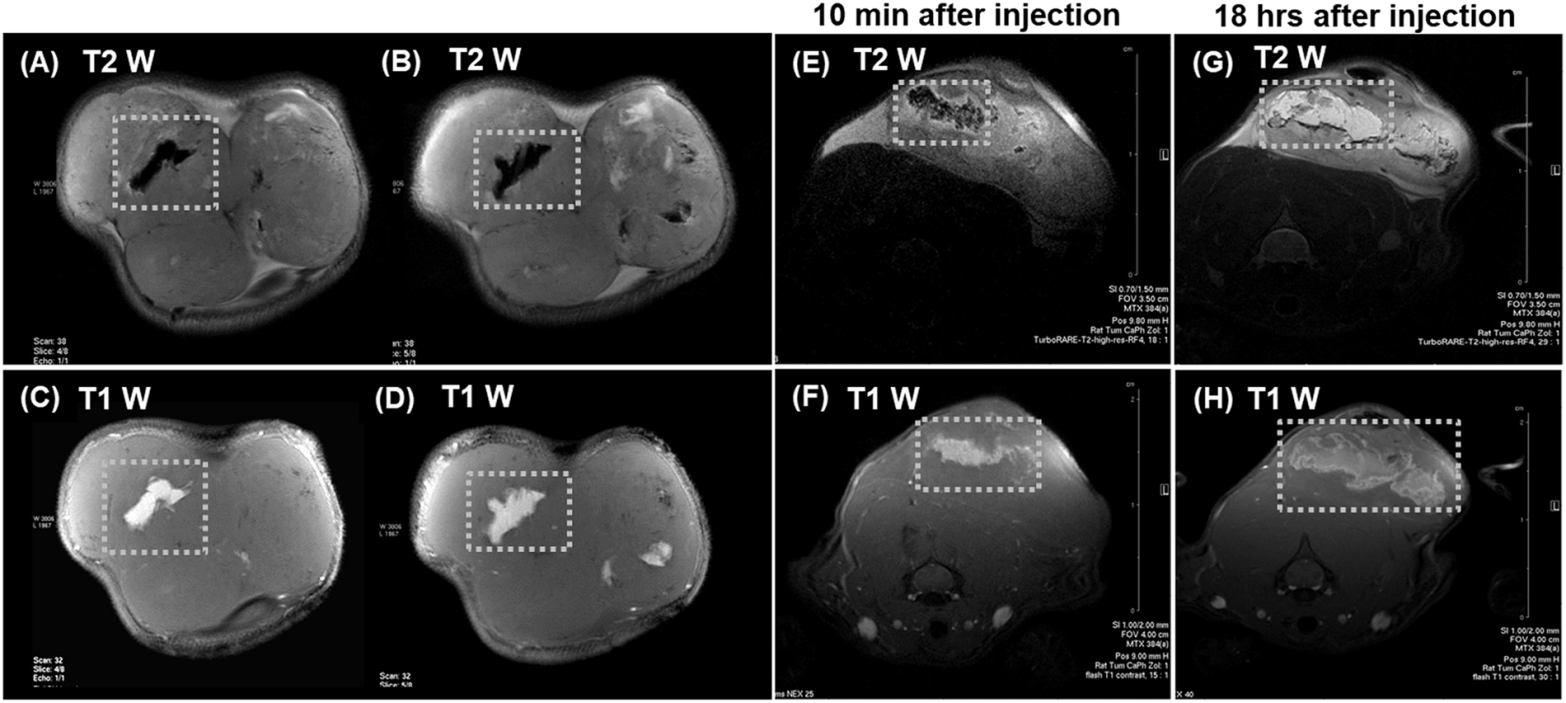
Dual T1-T2 contrast variation after intra-tumoral injection. (A,B) T2 weighted and (C,D) T1 weighted MR image (coronal section) of subcutaneous tumor after intratumoral nCP:Fe injection. (E) T2 weighted and (F) T1 weighted MRI of subcutaneous tumor (axial sections) 10 mins after intratumoral injection of nCP:Fe. (G) T2 weighted and (H) T1 weighted MRI of subcutaneous tumor (axial sections) 18 hours after intratumoral injection of nCP:Fe. Sample injected regions are shown in white dotted boxes.
